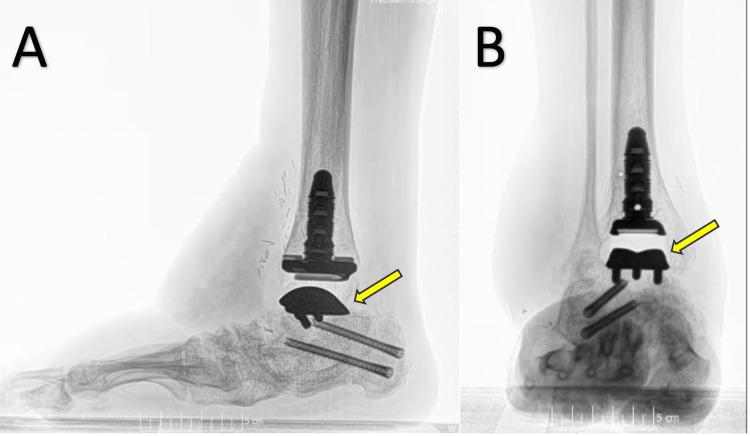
Radiographs of the patient’s right ankle three months postoperatively. (A) Lateral X-ray of the right ankle showing properly placed implants. (B) Anterior-posterior X-ray of the right ankle showing properly placed implants.
